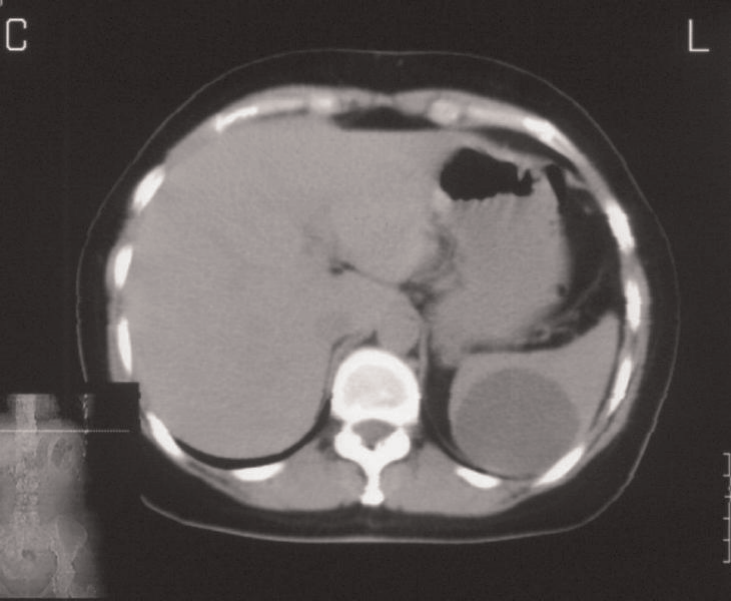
CT scan image showing a well-defined cystic lesion on the upper part of the spleen.
